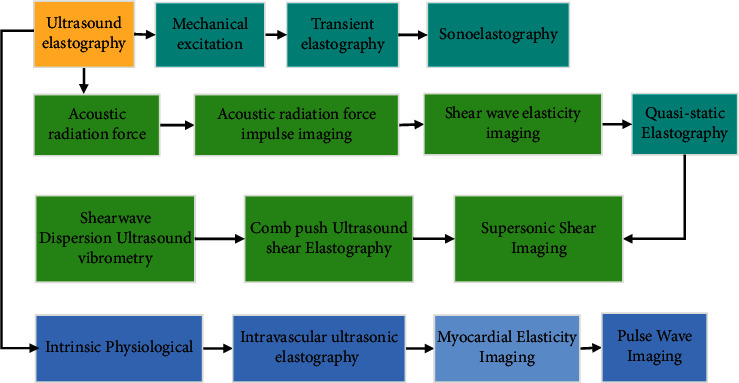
Classification and summary of ultrasonic elastography methods.
